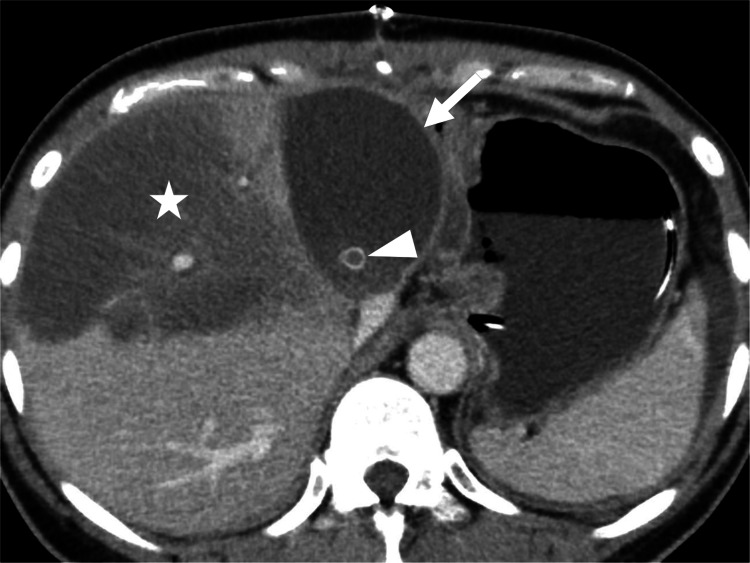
Contrast-enhanced CT of the upper abdomen showing a biloma (arrow) adjacent to the right lobe liver graft; the anterior portion of the liver shows hypodensity (star) due to occlusion of a segmental hepatic vein (arrowhead) that was reconstructed using an artificial graft.
